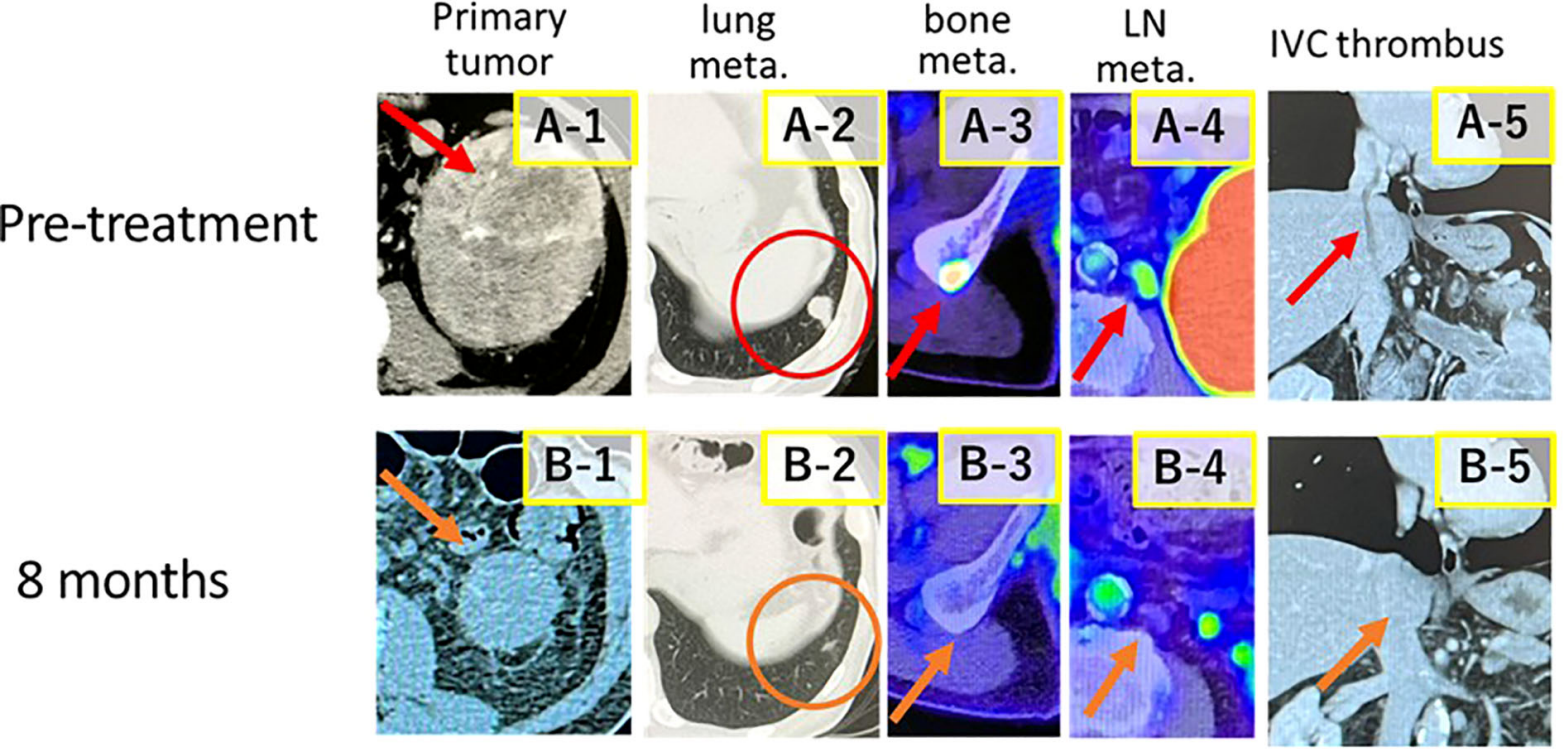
Computed tomography and positron emission tomography-computed tomography images (before treatment, red arrows; after treatment, orange arrows). Images of the primary tumor, lung metastases, bone metastases, lymph node metastases, and inferior vena cava (IVC) tumor thrombus. (A-1, A-2, A-3, A-4, and A-5). Images of the primary tumor and IVC tumor thrombi 8 months after combination treatment with ipilimumab and nivolumab are shown (B-1, B-2, B-3, B-4, and B-5).
